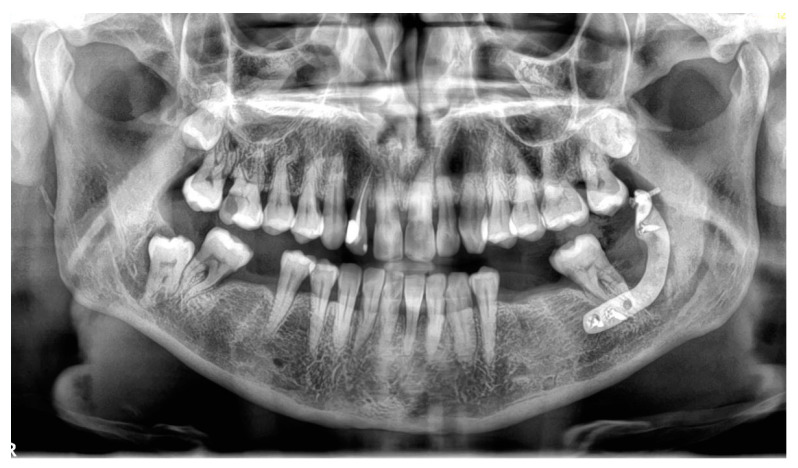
Post-surgical orthopantomography image.
